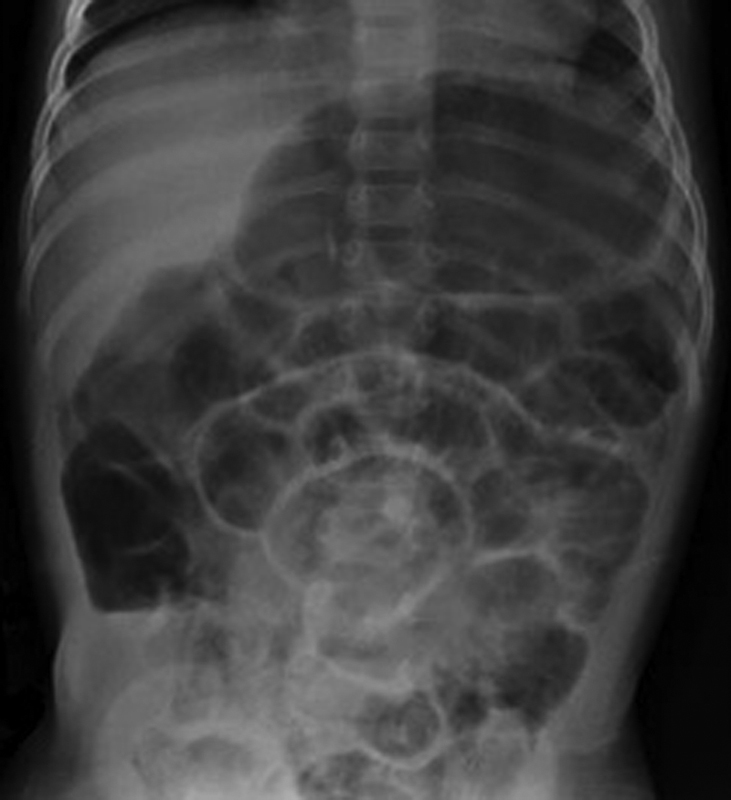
Abdominal X-ray. Right lower quadrant fixed air fluid level and small bowel dilatation.
